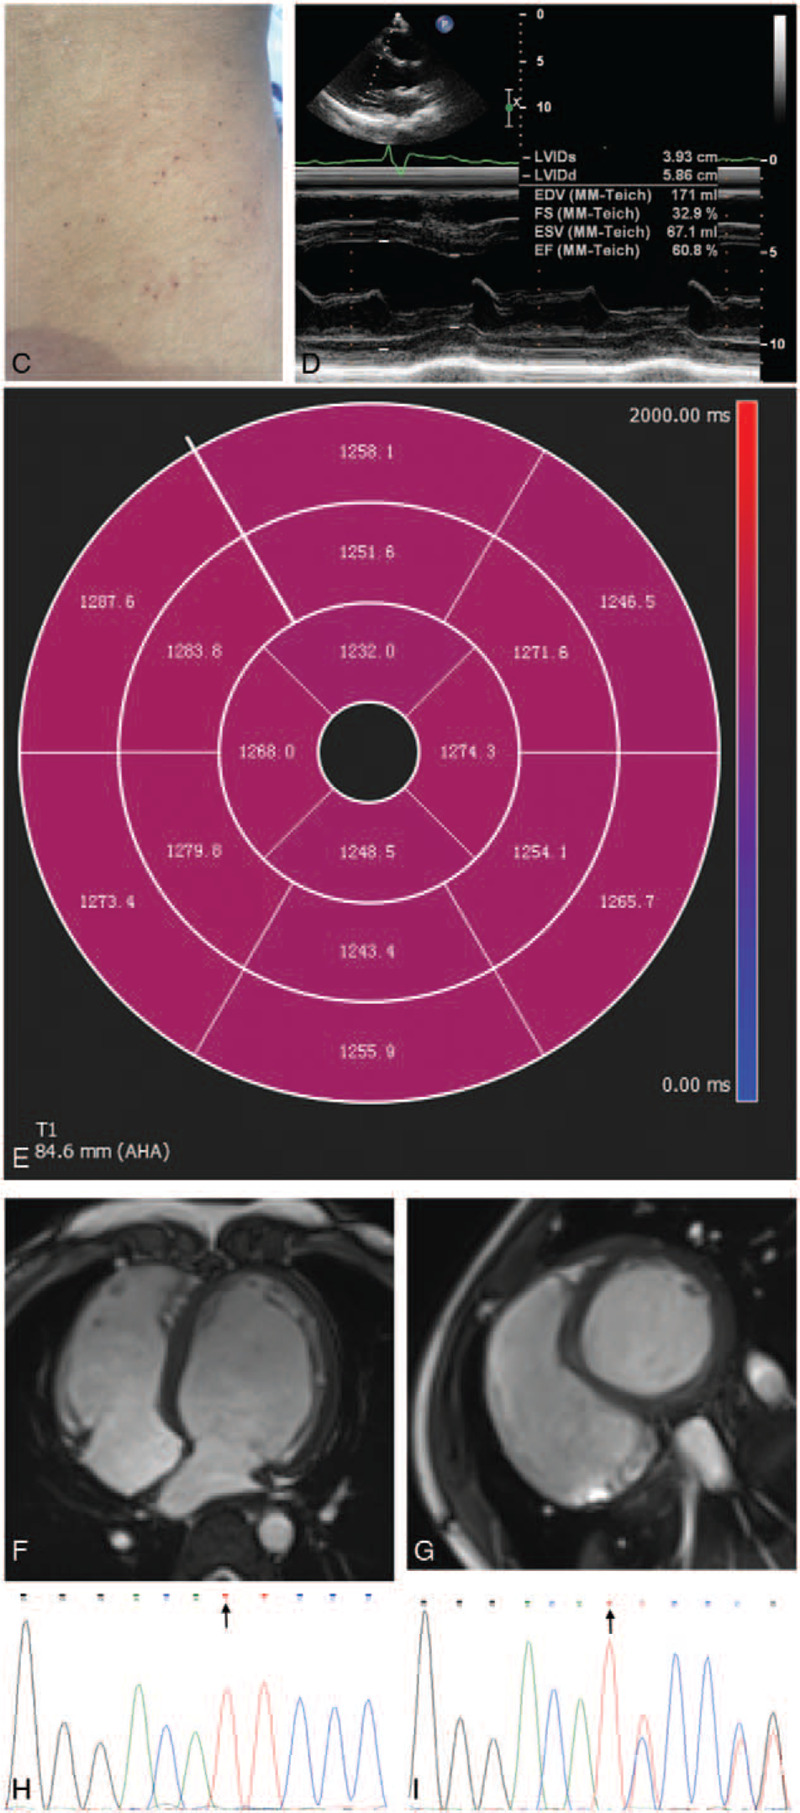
(A) I, II, III, aVR, aVL, aVF leads. (B) V1-V6 leads. (C) Sporadic angiokeratomas on the waist and back. (D) Transthoracic echocardiogram showing left ventricular dilation. LVIDs = left ventricular internal diameter in systole, LVIDd = left ventricular internal diameter in diastole, EDV = end-diastolic volume, ESV = end-systolic volume, FS = short-axis fractional shortening, EF = ejection fraction. (E) Native T1 mapping of the left ventricle. (F) Long-axis four-chamber CMR in diastole. (G) Short-axis two-chamber CMR in diastole. (H) Part of the electropherogram of exon 1 of the *GLA* gene in the patient. The mutation is indicated by the arrow. (I) The same part of the gene in the patients mother, in which the mutation is indicated by the arrow.
